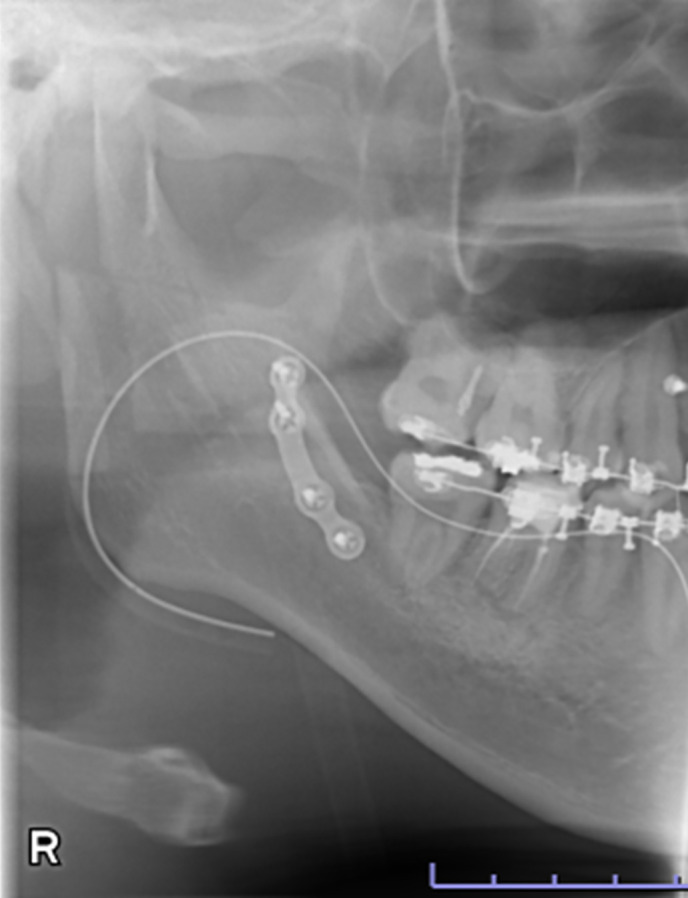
Panoramic radiograph showing the lateral osteotomy line in Trauner–Obwegeser method.
